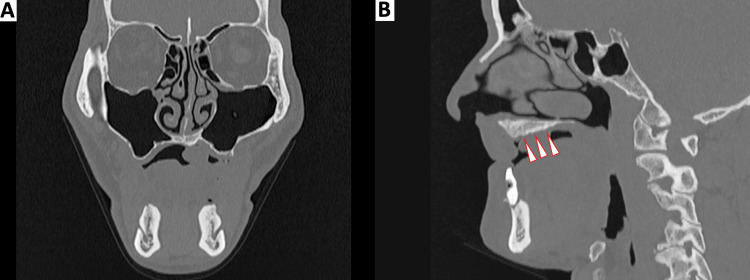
Computed tomography (CT) findings of the palatal lesion A) Coronal CT image showing a soft-tissue mass on the right anterior hard palate without cortical erosion of the palatal bone. B) Sagittal CT image confirming the absence of underlying bony resorption beneath the lesion; the arrow indicates the intact palatal bone surface.
